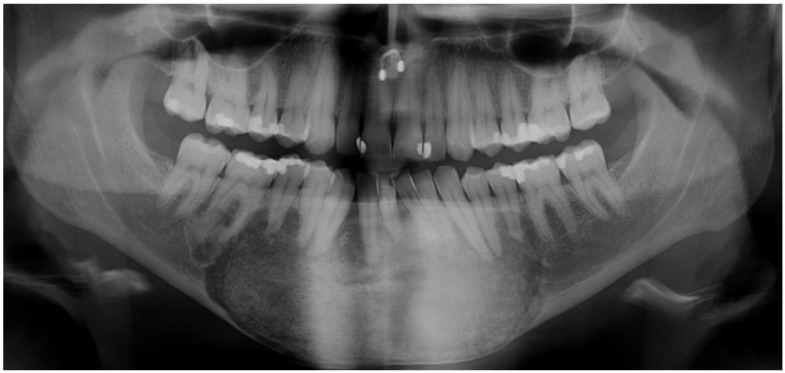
Panoramic radiography of FD in the anterior mandible. The characteristic features of FD of the jaw, such as ground-glass appearance and thin borders, are represented.
